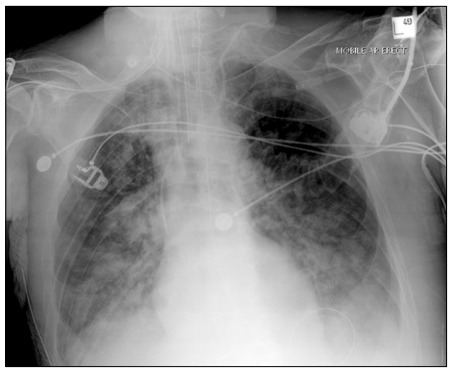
Chest X-ray used for COVID-19 training. Case courtesy of Prof Frank Gaillard, Radiopaedia.org.
